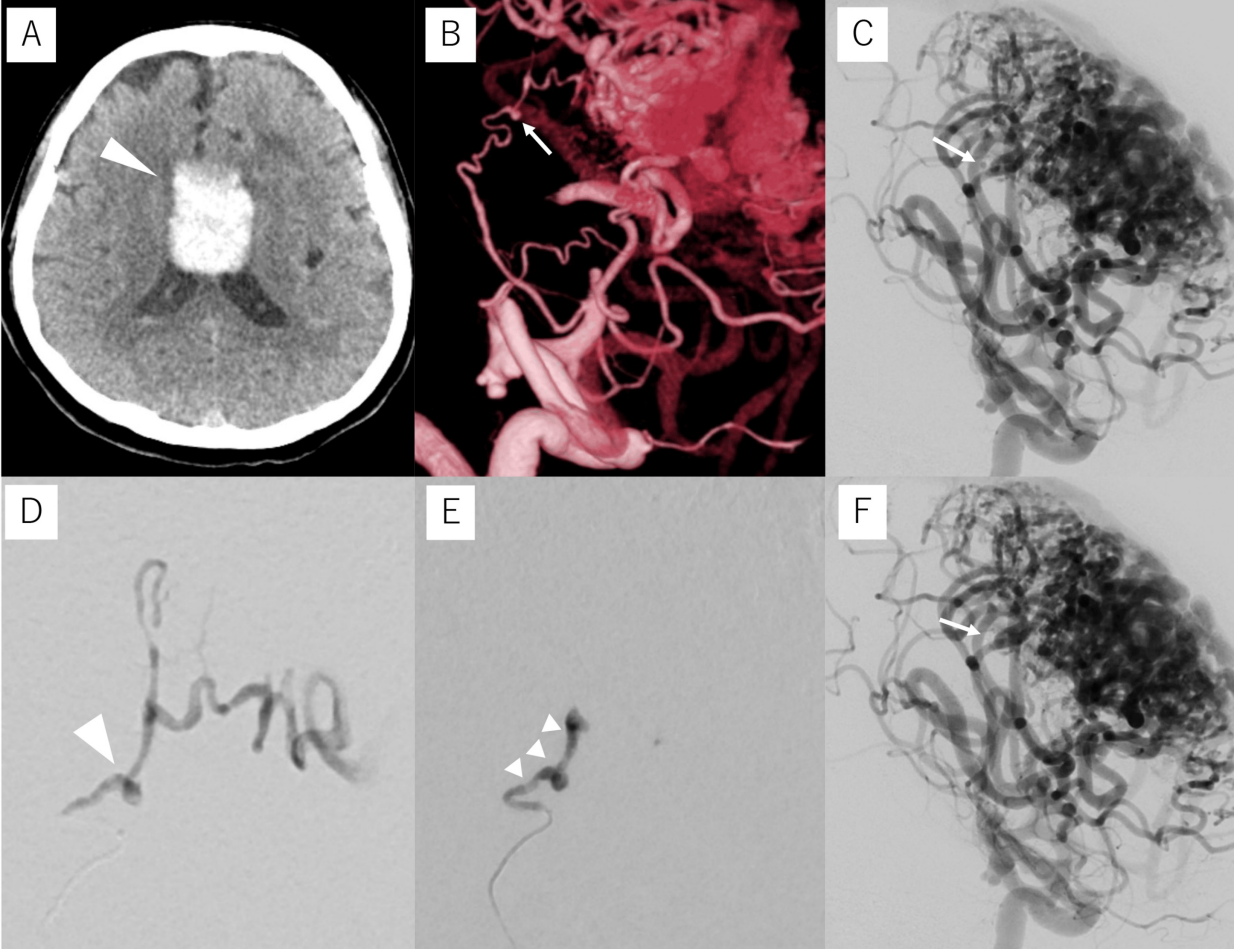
Preoperative and intraoperative imaging of Case 4 Case 4: A 64-year-old male with an intracerebral hemorrhage near the corpus callosum. (A) Computed tomography (CT) upon admission shows a cerebral hemorrhage near the corpus callosum (arrowhead). (B, C) Digital subtraction angiography (DSA) of the left internal carotid artery shows AVM and a 1.2-mm fusiform aneurysm (arrow) on a lenticulostriate artery. (D) Selective angiography of the parent artery (working-angle view) shows a fusiform aneurysm (arrowhead). (E) The aneurysm and parent artery are embolized with 33% N-butyl cyanoacrylate glue (arrowheads). (F) Post-embolization DSA of the left internal carotid artery shows the disappearance of the aneurysm (arrow).
